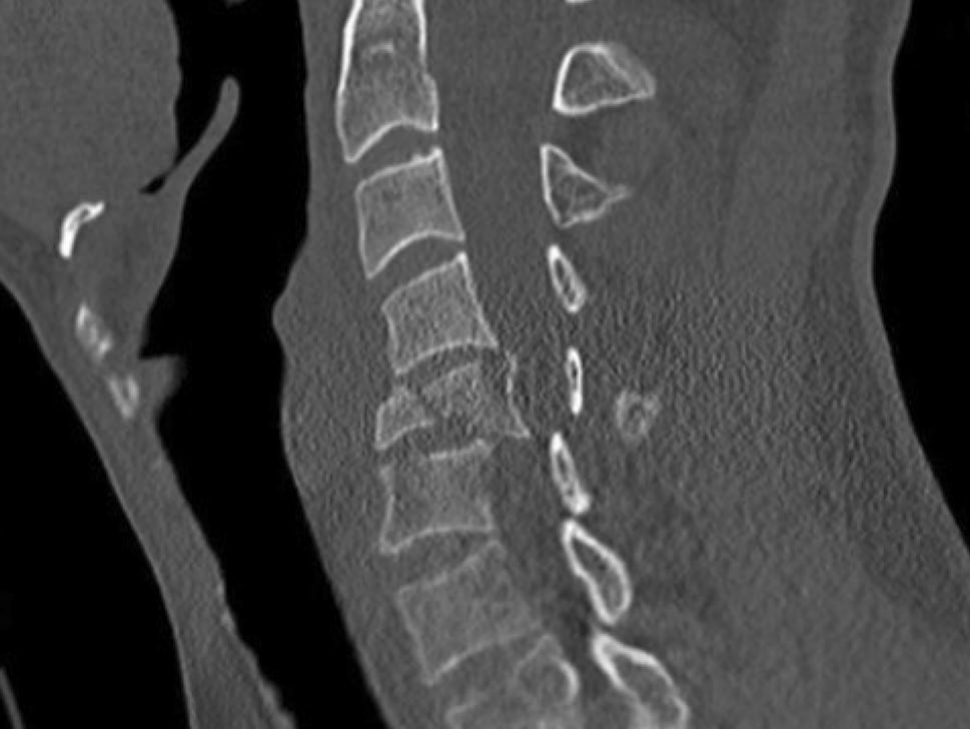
Burst fracture, CT scan, sagittal view
